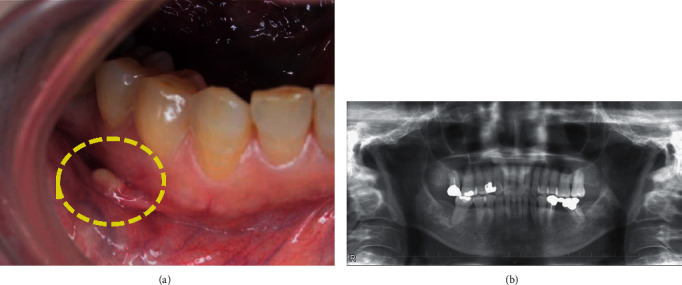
Intraoral clinical findings and imaging findings of the 76-year-old female patient. (a) A whitish-colored submucosal mass covered with normal mucosa was observed on the mucosal aspect of the right lower lip. The *dotted line* indicates the extent of the lesion. This lesion was 5 mm in max. Dia. and was clinically diagnosed as a benign tumor. (b) Dental panoramic radiography provided no significant findings that seemed to be associated with the mass.
